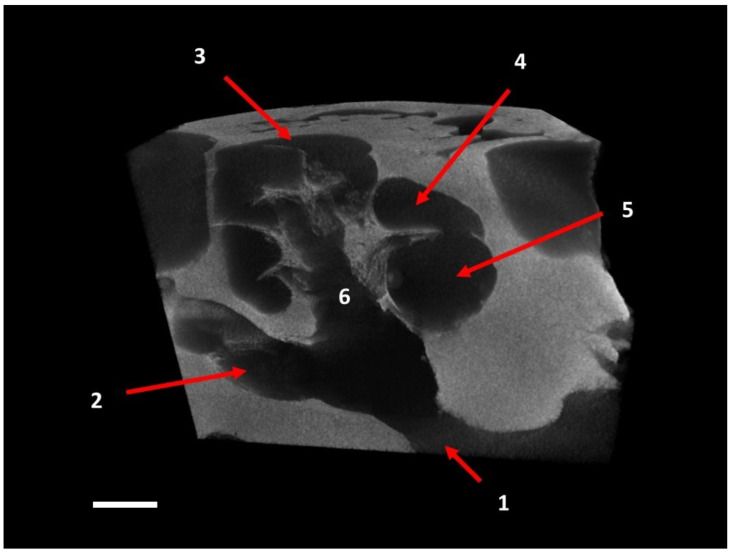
Vertical cross section of the cochlea at level of the modiolus. 1, internal acoustic meatus; 2, beginning of the scala tympani near the round window; 3, last turn; 4, scala vestibuli; 5, scala tympani; 6, bony cavity of the modiolus housing the cochlear nerve (3D reconstruction by µ-CT projections). Scale bar = 1 mm.
